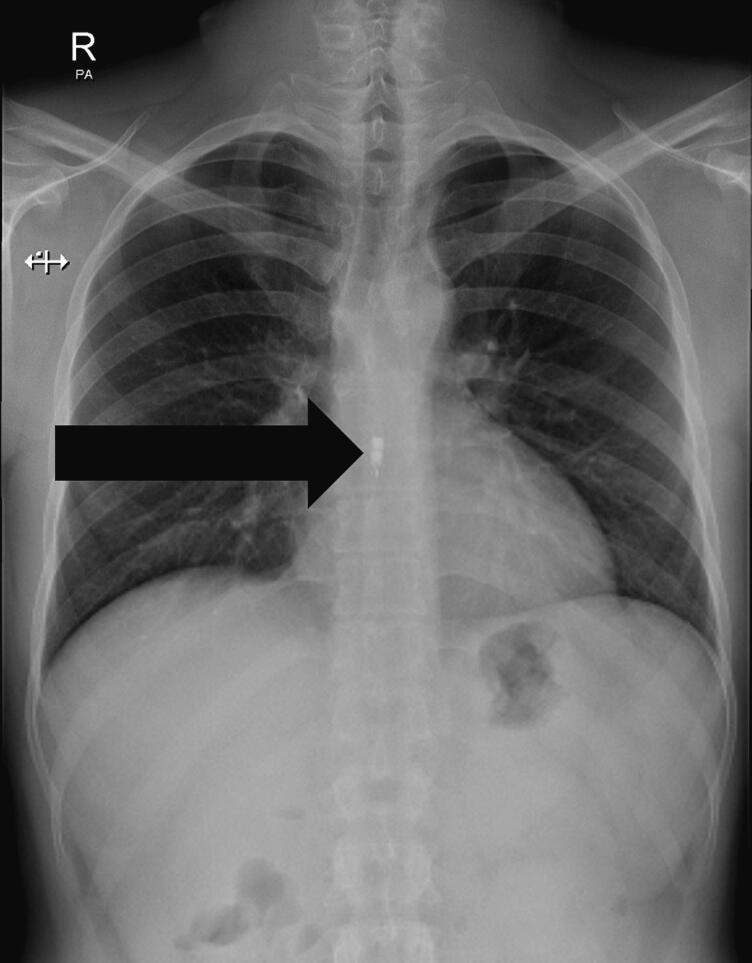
Chest X-Ray showing retention of oesophageal pH capsule with arrow pointing to retained capsule.
